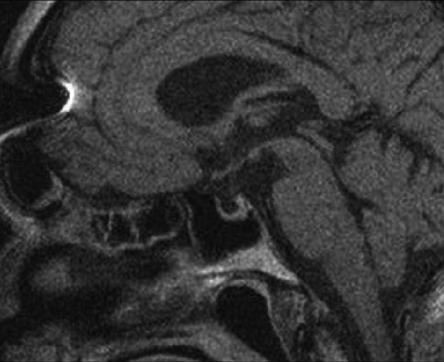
Sagittal MRI view of hypothalamic-pituitary area showed reduction of pituitary parenchyma.
